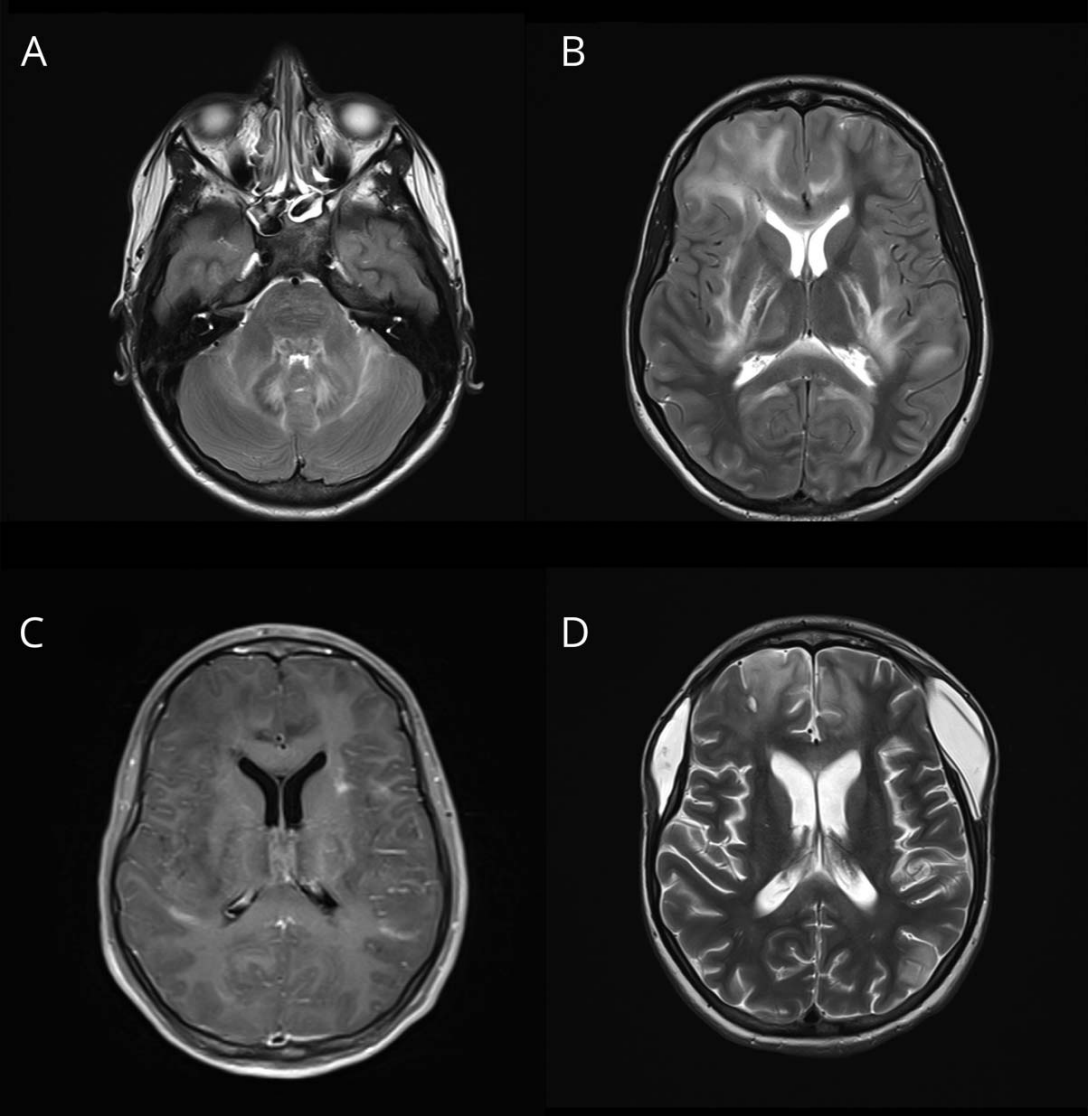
Representative MR images Representative neuroradiologic images. (A–C) Acute imaging on day 2 of admission shows bilateral, asymmetrical, predominantly white matter changes, although some gray matter involvement of thalami is also seen. Patchy enhancement postcontrast and mass effect and effacement of the sulci. Diffusion-weighted imaging (not shown) did not indicate any area of restricted diffusion. (D) Approximately 1 month later showing postcraniectomy changes and substantial resolution of the acute inflammation. (A, B, and D = T2-weighted; C = postcontrast T1-weighted).
